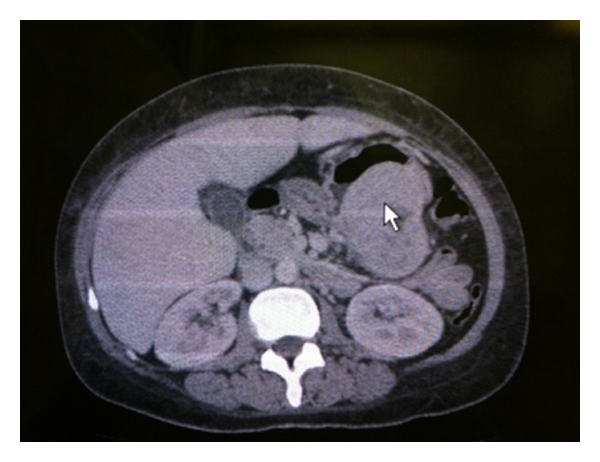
CT scan showing the lesion in the posterior wall of stomach (arrow).
